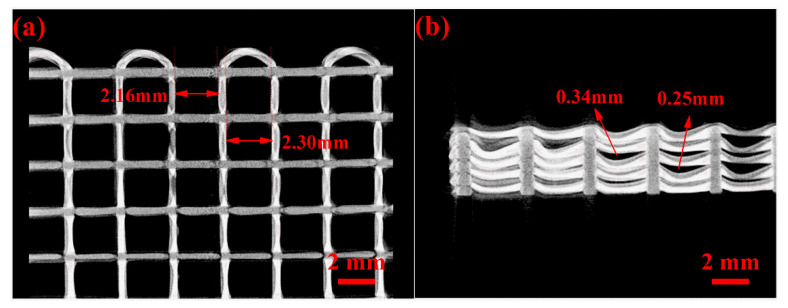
Computed tomography (CT) characterization images of silicone foams: (a) top view and (b) side view.
